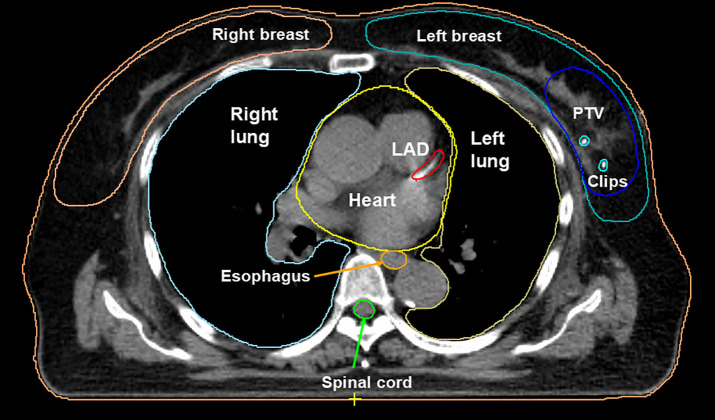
Axial views of the PTV and OARs contoured using the planning CT image. PTV, planning target volume; OARS, organs at risk.
